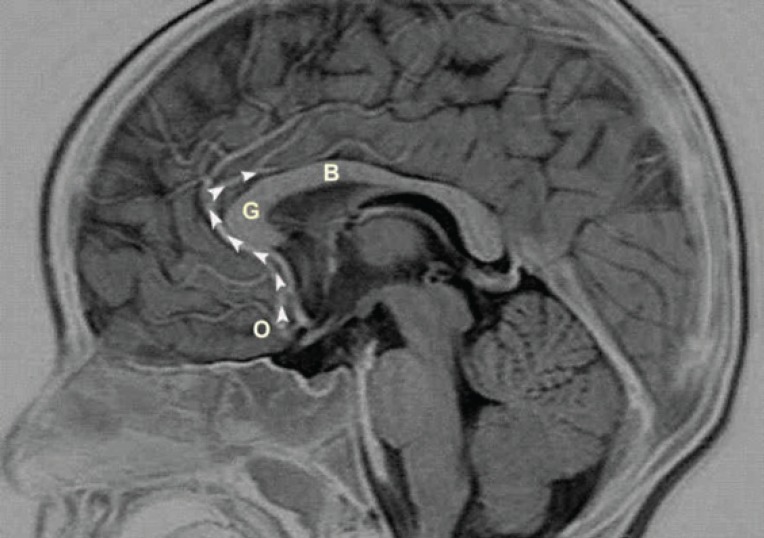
Mid-sagittal T2-weighted negative picture of cranial magnetic resonance image. The length of anterior cerebral artery has been measured from origo of anterior cerebral artery to junction of genu and body of corpus callosum (white arrows). B: Body of corpus callosum, G: Genu of corpus callosum, O: Origo of anterior cerebral artery
